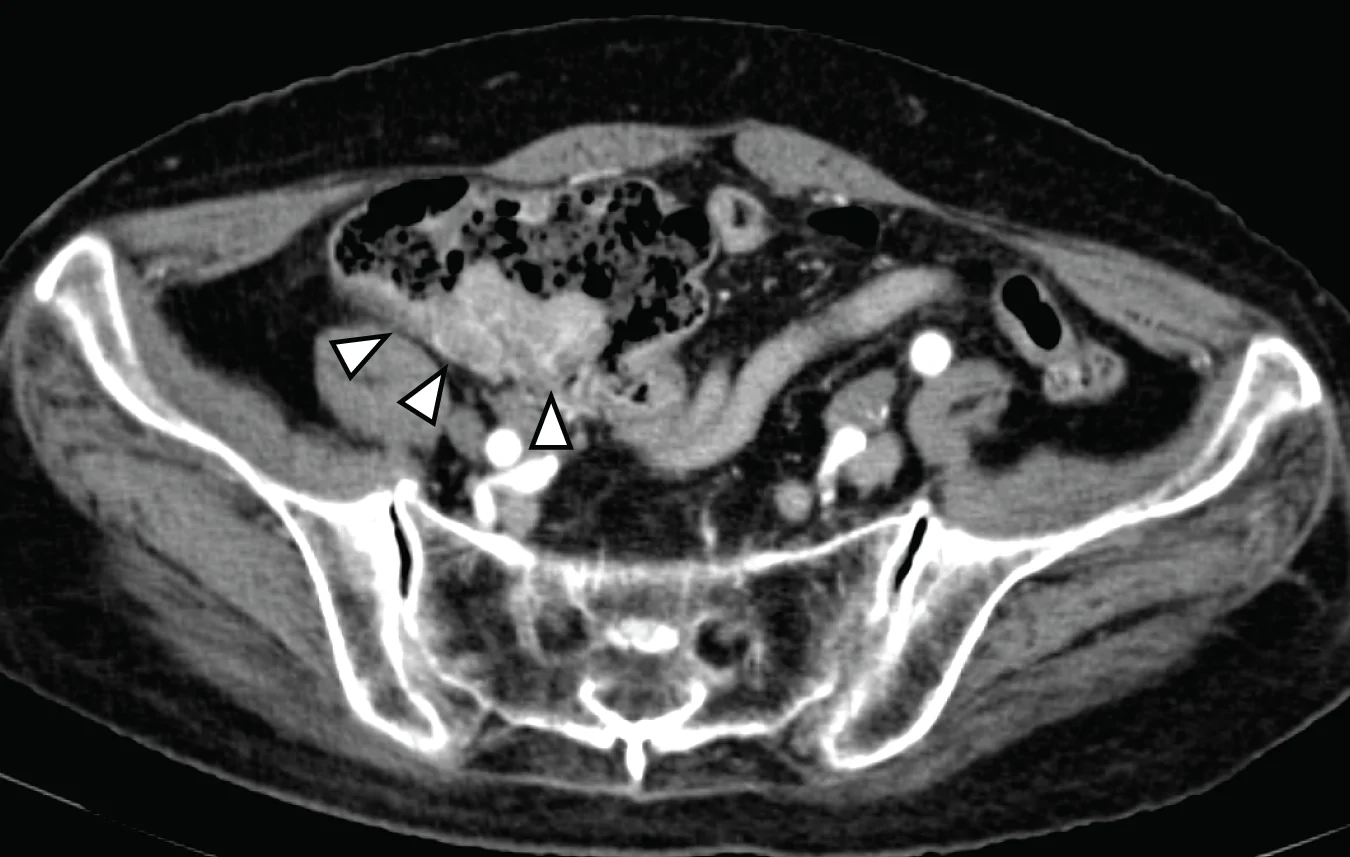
Abdominal contrast-enhanced CT showed wall thickening with contrast enhancement in the ascending colon (arrowhead). No lymph node enlargement was recognized.
